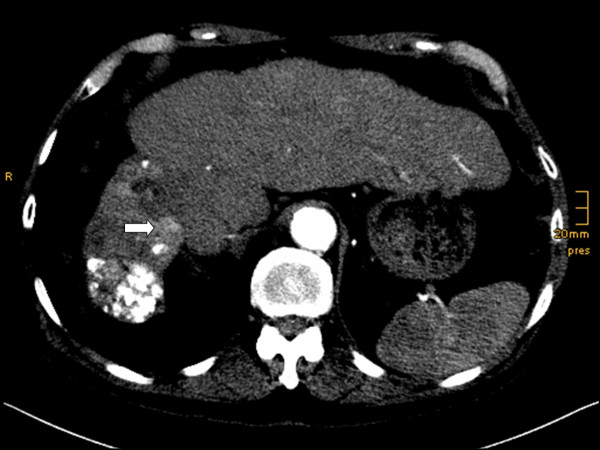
Baseline CT abdomen scan. Early arterial examination time, showing liver cirrhosis after transarterial chemoembolization with hypotrophy of his right liver lobe and hypertrophy of his left liver lobe. Lipiodol remnants in the right lobe. Arrow: TL1 in segment 8, size 20 mm.
